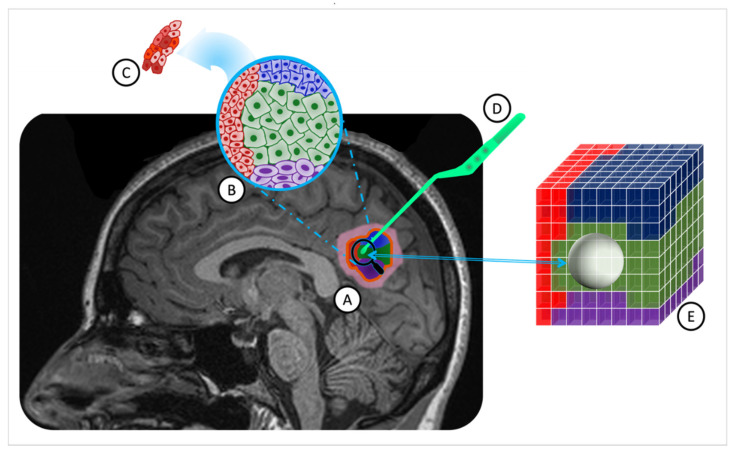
ITH in a solid brain tumor and MRI-guided stereotactic biopsy overlaid on a T1_W_ MR image. (A). A solid brain tumor outlined by a yellow border, comprising four distinct cell subpopulations (in red, blue, green, and purple) with associated inflammation in pink. (B). Macroheterogeneity within cell subpopulations, displaying reduced cytoplasm and enlarged nuclei in some cells. (C). Microheterogeneity among tumor cells of the same subpopulations. (D). The pituitary tweezer is used for stereotactic biopsy. (E). The tumor sample volume is represented as a sphere, centered on the biopsied location, including two cell populations (blue and green). (ITH: intratumoral heterogeneity; MRI: magnetic resonance imaging; T1_W_: T1-weighted; MR: magnetic resonance).
